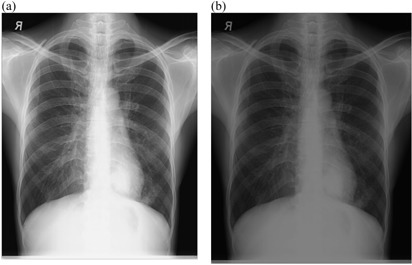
Low kVp chest radiograph: (a) original image and (b) processed image by linear spatial filtering.
